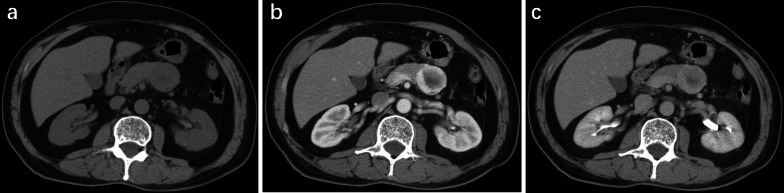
NET of the pancreatic body in a 72-year-old man. a Unenhanced CT scan shows a well-defined solid and cystic mass with a size of 3.5 cm. The CT value of the tumor is 41 HU. b Arterial phase CT scan shows marked heterogeneous enhancement of the lesion with a rim type. The CT value of the tumor is 133 HU. c Portal phase CT scan shows areas of washout. The CT value of the tumor is 98 HU
